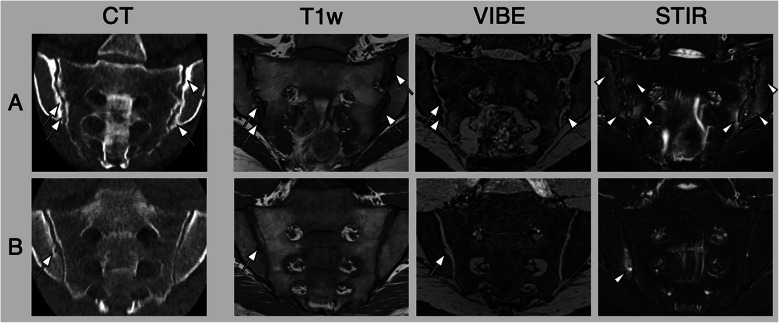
Low-dose CT in patients with axial spondyloarthritis. Both scans were conducted utilizing a volume scan technique without table movement, employing parameters of 120 kVp and 5 mAs. This methodology resulted in a dose length product of 2.6 mGy*cm and a computed tomography dose index volume (CTDIvol) of 0.3 mGy, with an estimated effective dose of less than 0.04 mSv. The reconstruction of the scans was performed using artificial intelligence algorithms. Patient A displays advanced sacroiliitis characterized by bilateral erosion and sclerosis (white arrows) along with bone marrow edema (white arrowheads). Conversely, patient B exhibits only mild erosion (white arrow) and bone marrow edema (white arrowhead)
